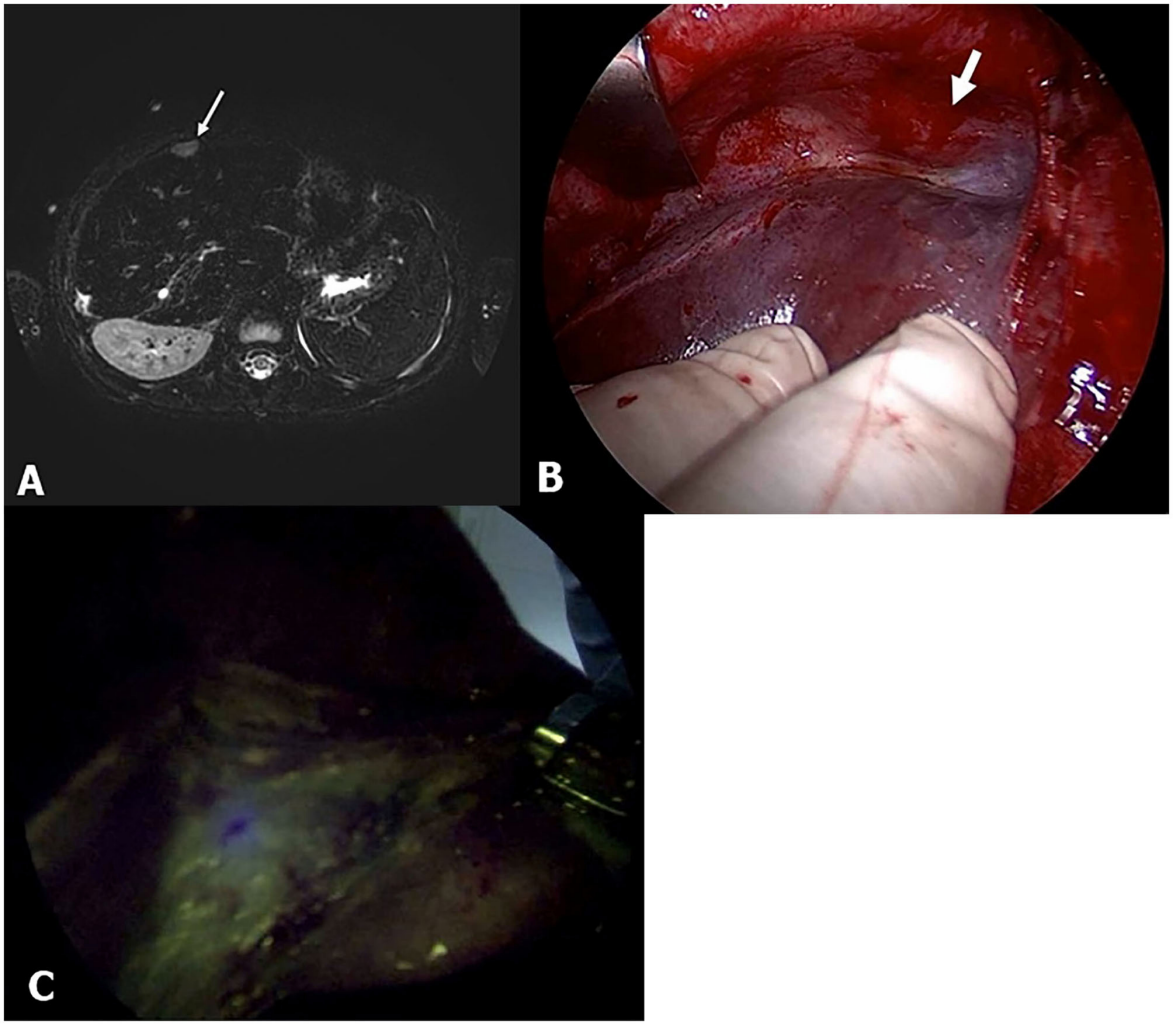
Pt. No. 10 in Table 2: (A) T2-weighted magnetic resonance (MR) showed the supradiaphragm metastatic lesion (white bold arrow). (B) Without fluorescent imaging. (C) Fluorescent imaging enabled visualization of the metastasis of HB that was palpable but clearly unidentified in gross.
